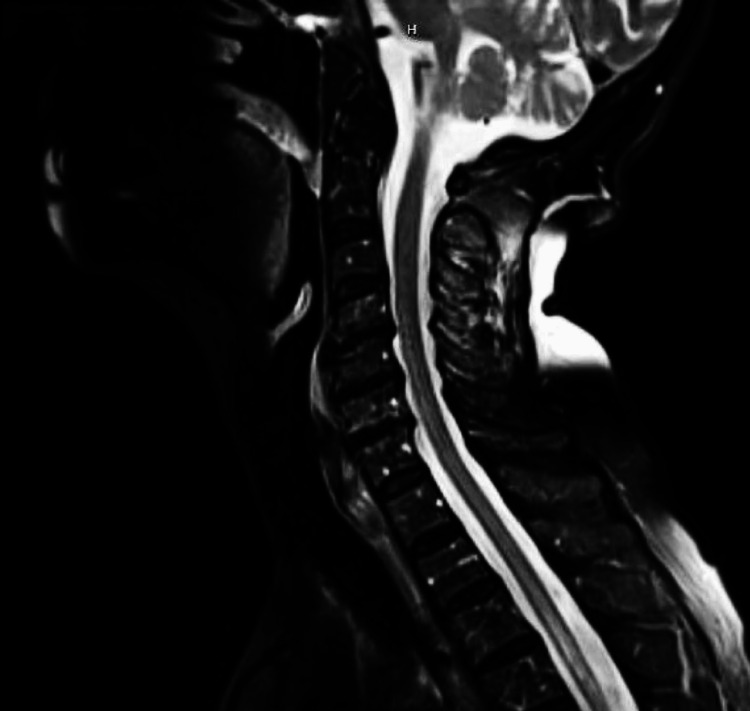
Repeat T2-weighted cervical spine MRI on day 8, sagittal view, showing nearly complete resolution of increased T2 signal at the C7 level MRI: magnetic resonance imaging
